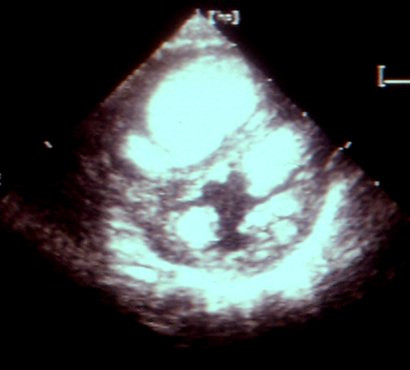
Echocardiography on a newborn child shows multiple rhabdomyomas in both ventricles. In this child the diagnosis of tuberous sclerosis was confirmed.
